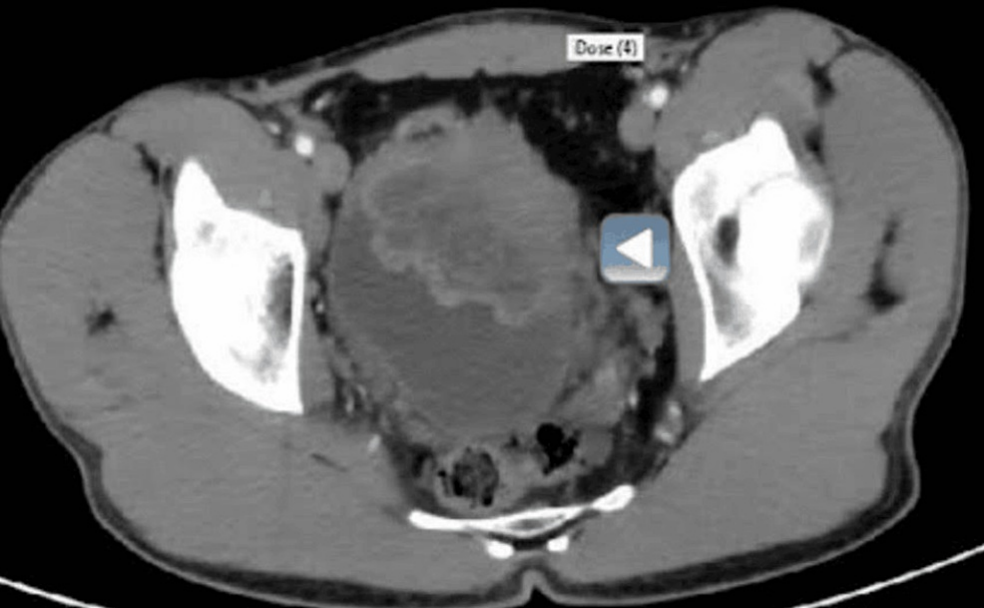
Enhanced CT scan of the abdomen and pelvis showing a large anterior urinary bladder mass lesion with luminal filling of the anterior compartment of the urinary bladder and mural involving the anterior wall extending beyond the urinary bladder, measuring about 7.5 × 6 × 6 cm in the mediolateral, anteroposterior, and craniocaudal dimensions, respectively.
